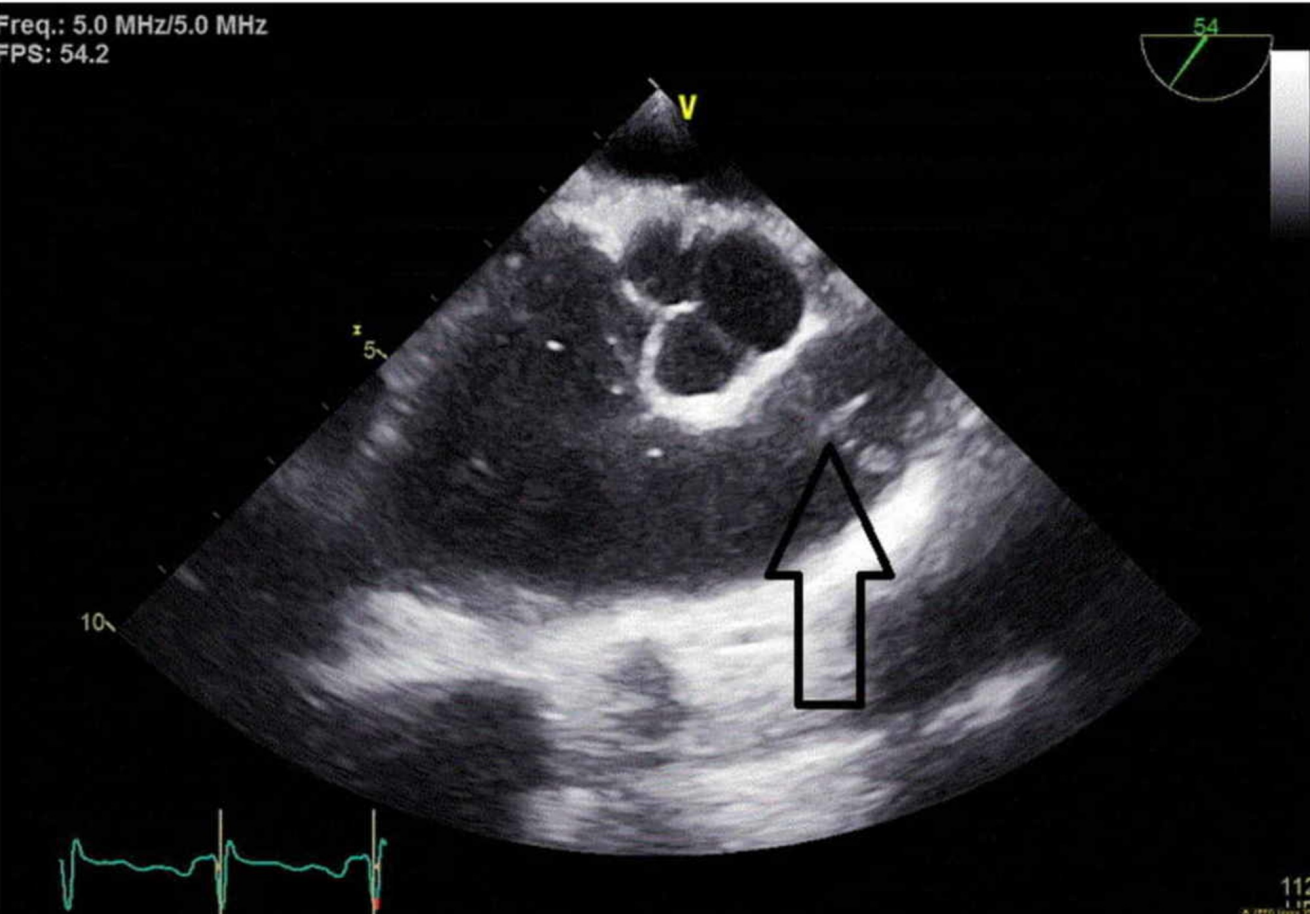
Transesophageal echocardiogram. Resolution of vegetation after intravenous therapy.
